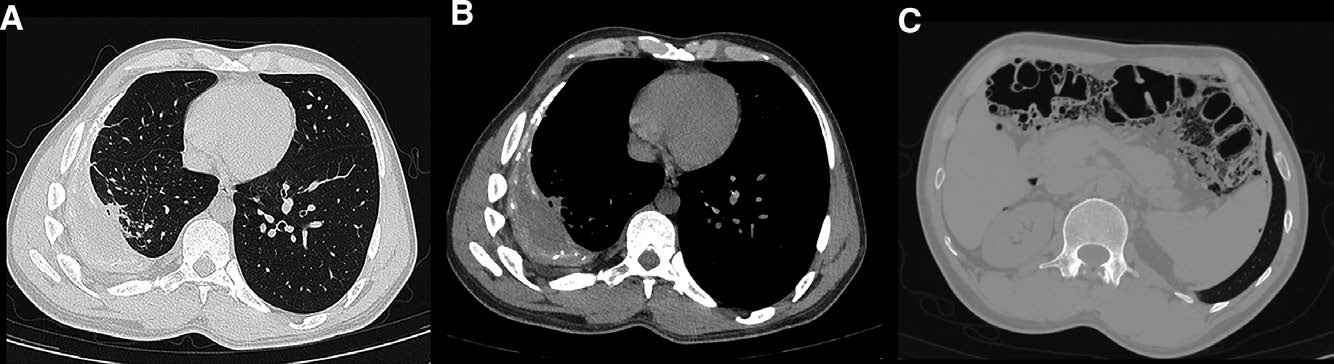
August 20, 2024, CT: (A) lung window and (B) mediastinum window revealed encapsulated pleural effusion in the right thoracic cavity, along with pleural thickening and calcification on the right side, (C) PCI with pneumoperitoneum. CT = computed tomograph, PCI = pneumatosis cystoides intestinalis.
